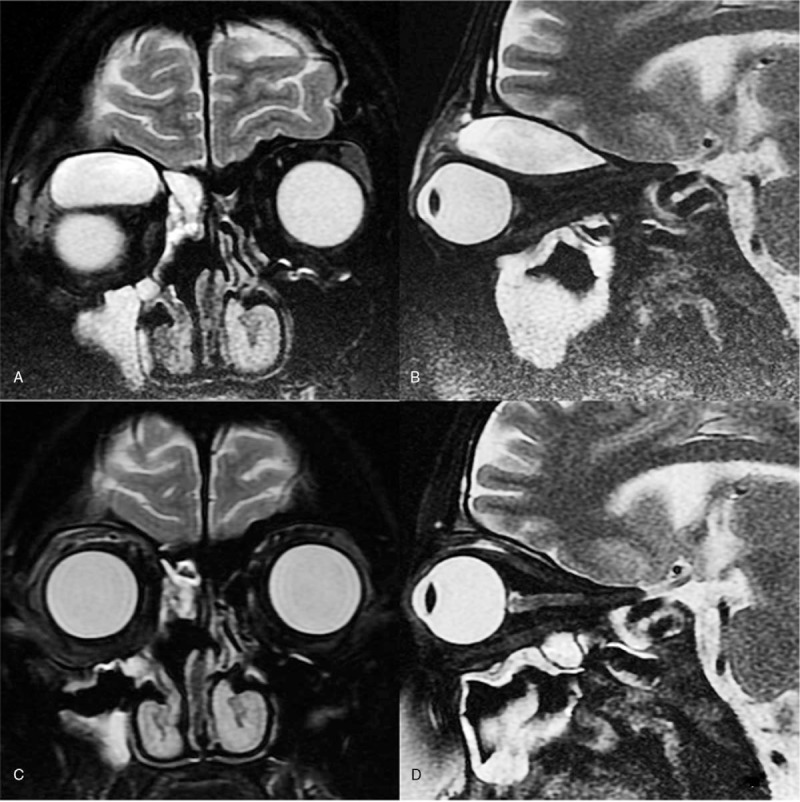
(A) Postoperative coronal T2-weighted magnetic resonance image showing that the right orbital abscess is pushing the eyeball forward and downward. (B) Postoperative sagittal T2-weighted magnetic resonance image showing that the right superior orbital abscess is pushing the eyeball forward and downward. (C) Coronal T2-weighted magnetic resonance image showing that the right orbital abscess has disappeared after ultrasound-guided fine needle aspiration and catheter drainage; the eyeball has returned to the normal position. (D) Sagittal T2-weighted magnetic resonance image showing that the right orbital abscess has disappeared after ultrasound-guided fine needle aspiration and catheter drainage; the eyeball has returned to the normal position.
